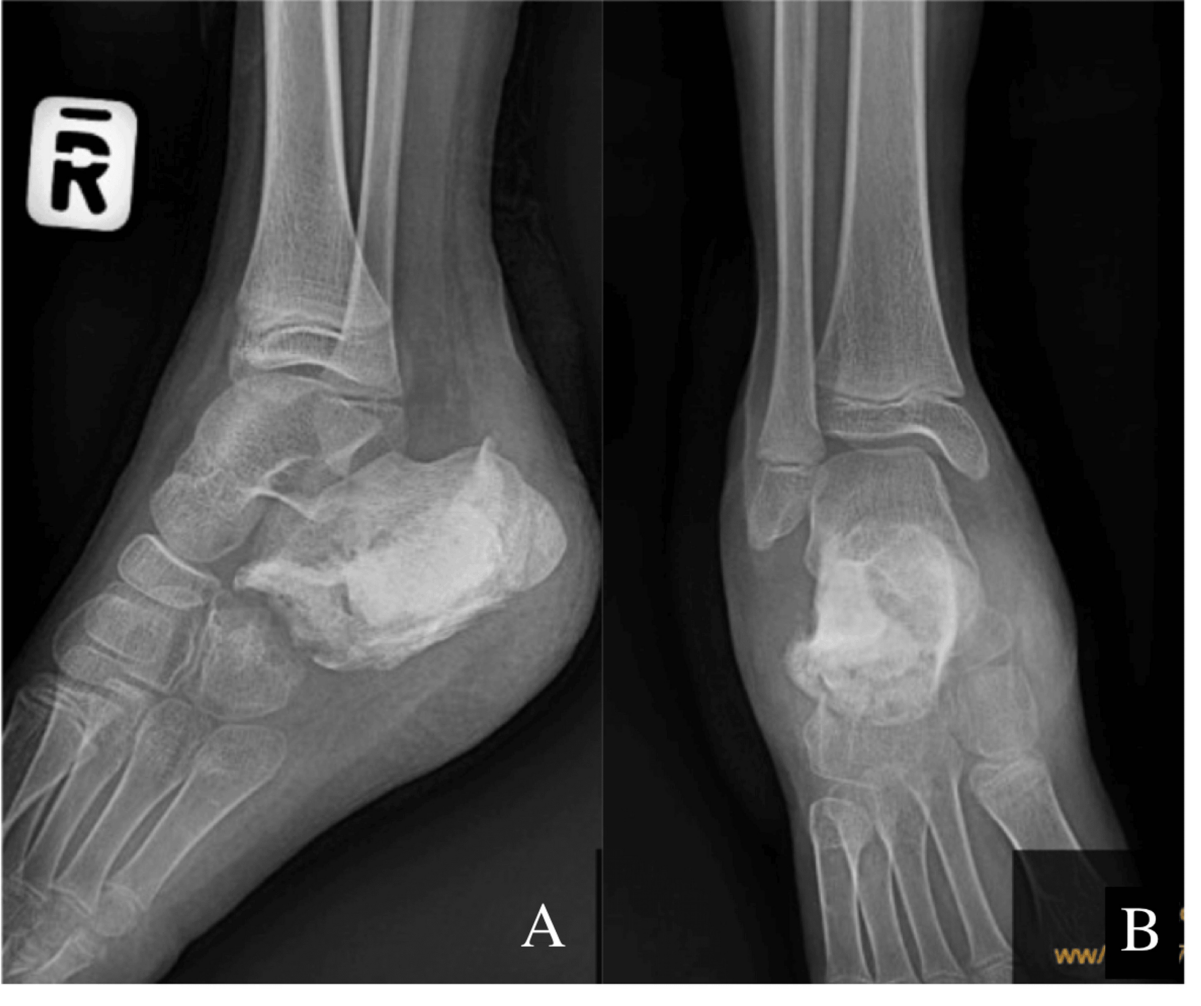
Postoperative radiographs with antibiotic bone cement filling the cavity showing in lateral (A) and anteroposterior (B) view
